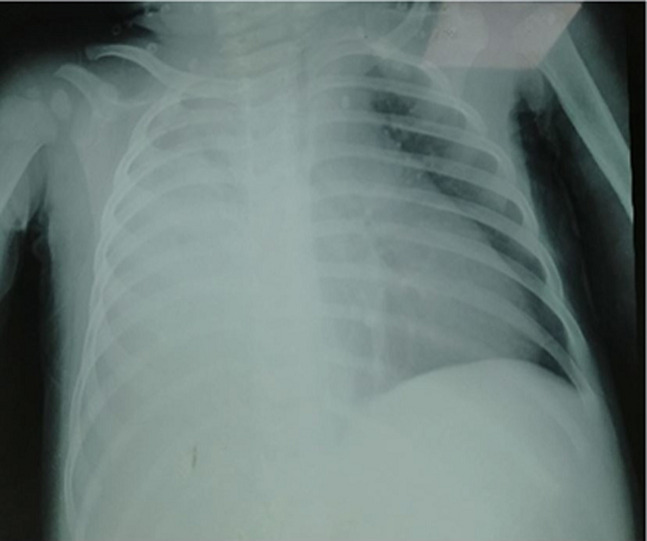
the initial chest X-ray showing an effusion and pneumonia on the right with a mediastinal widening and a displacement of the heart to the left
